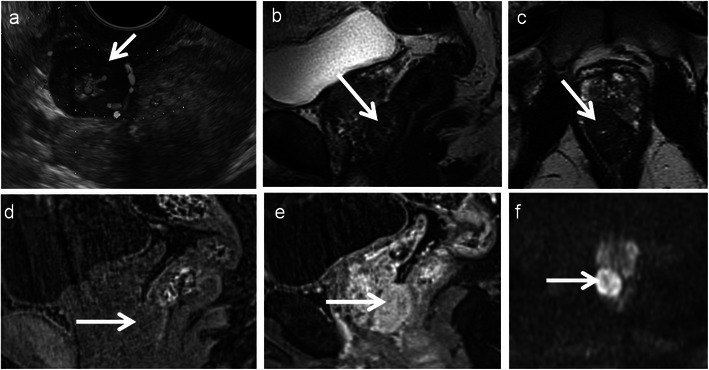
Rectal GIST in a 55-year-old man with a palpable mass on digital rectal examination. a Transrectal ultrasound reveals a hypoechoic vascular mass (*arrow*) arising from the rectal wall. b Sagittal and c axial T2-weighted MRI reveals the lesion (*arrow*) to be heterogeneously hypointense and is arising exophytically from the right anterolateral wall. d Sagittal fat-suppressed T1-weighted MRI reveals the lesion (*arrow*) to be intermediate signal intensity and e contrast-enhanced T1-weighted MRI reveals intense enhancement (*arrow*). f The mass is bright on diffusion-weighted (*b*-1,000) MRI
